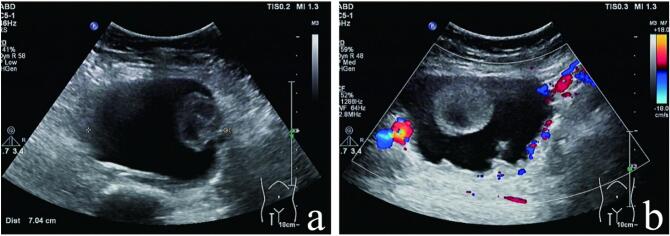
(A) Two-dimensional ultrasonography of a mixed echogenic mass in the muscular layer of the right thigh: A two-dimensional ultrasonography revealed a mixed echogenic mass measuring 8.5 × 7.3 × 5.3 cm in the muscularis propria of the middle and lower right thigh. The mass had clear boundaries but displayed an irregular morphology. It appeared mostly anechoic with an irregular wall. Additionally, a rounded and slightly strong echogenic mass measuring approximately 3.5 × 2.2 cm was observed attached to the capsule; (B) Color Doppler flow imaging of a right thigh mass: the mass did not exhibit any blood flow signal on color Doppler flow imaging, but peripheral blood flow signals were observed in dots and stripes.
